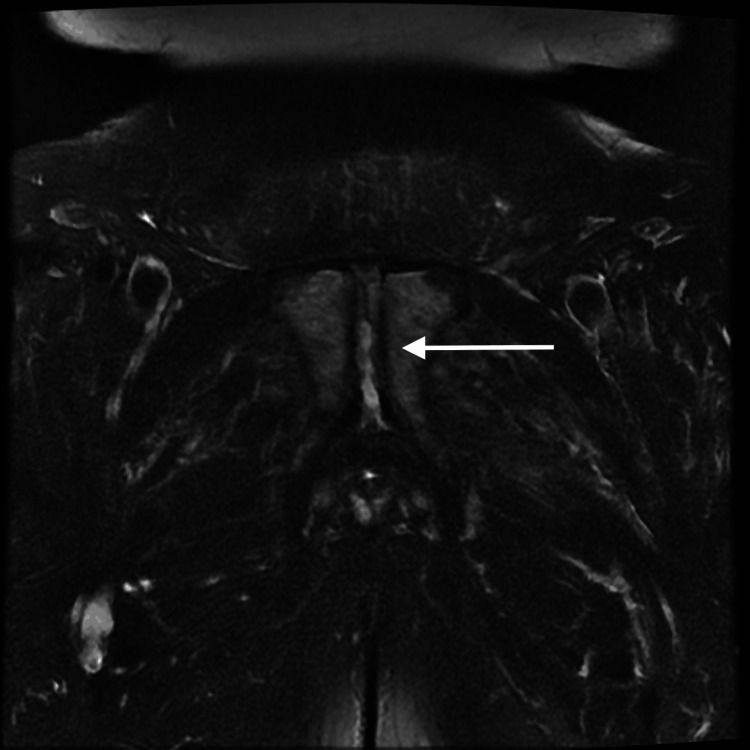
MRI at the initial presentation. The axial view shows a fistula tracking from the prostate coursing anteriorly to the symphysis pubis. There is an increased signal of the symphysis pubis, in keeping with osteomyelitis. MRI: magnetic resonance imaging
